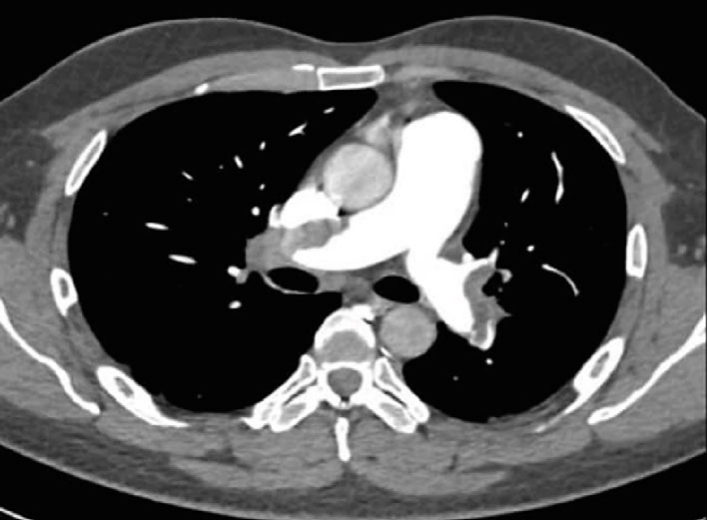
CT angiography of pulmonary artery (CTPA) revealed large filling defects in the right and left pulmonary arteries extending into segmental and subsegmental branches.
